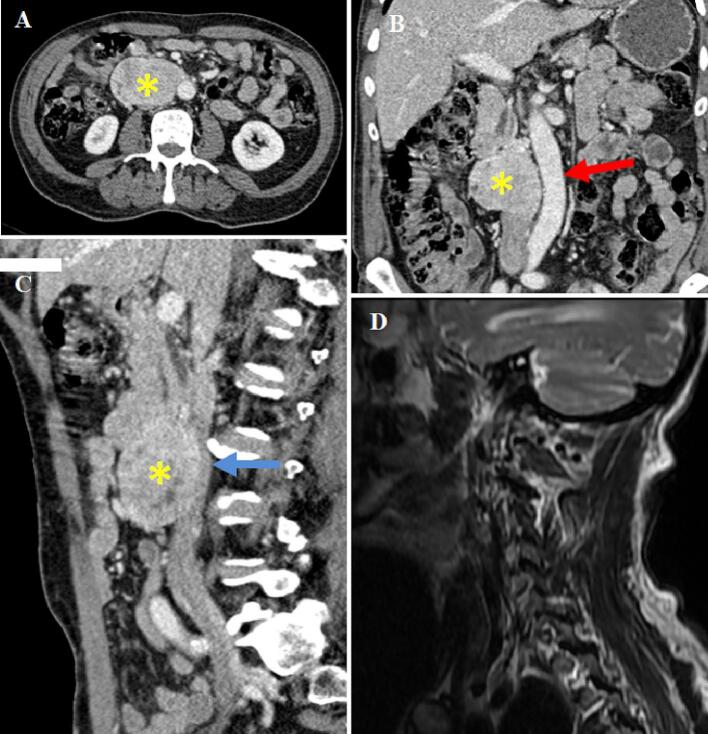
Imaging findings: contrast CT: an axial image of the portal venous phase (A), coronal image (B), sagittal image (C), the retroperitoneal mass of 8×7×3 cm with homogeneous aspect and hemorrhagic remodeling (*), backflowing the abdominal aorta (red arrow), and compressing the IVC (blue arrow); T2 weighted sagittal MRI image of the skull and the neck showing the absence of metastasis in this stage (D). (For interpretation of the references to color in this figure legend, the reader is referred to the web version of this article.)
